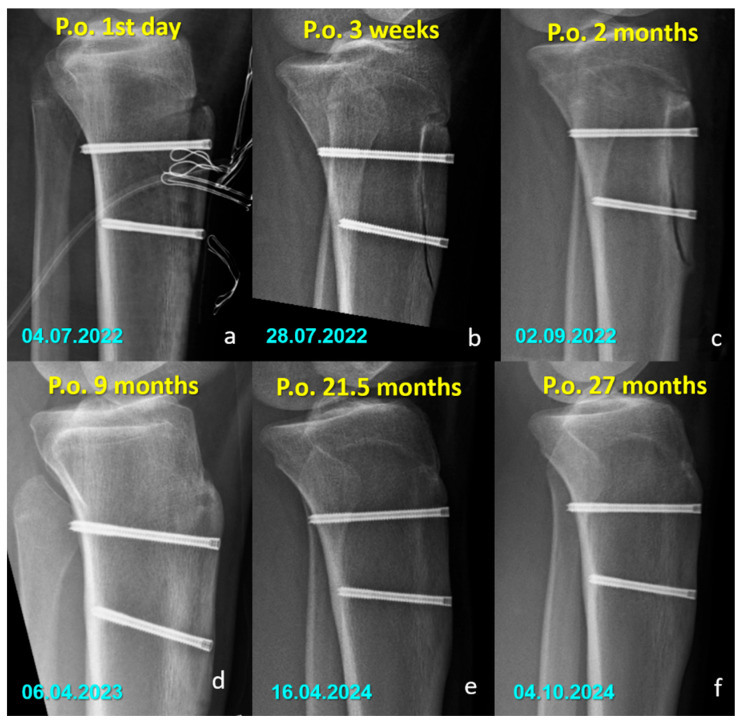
Serial lateral radiographs (a–f) showing the postoperative course of a patient treated with titanium compression screws following Fulkerson osteotomy.
